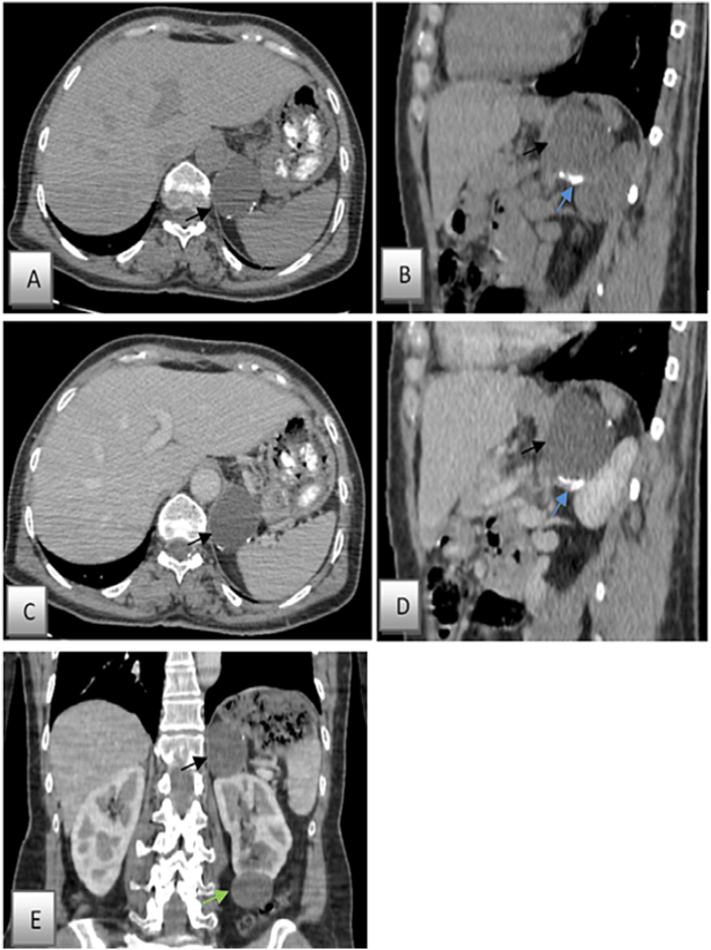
Non contrast-enhanced abdominal CT scan images (Fig. A: axial, Fig. B: sagittal), and non enhanced images (Fig. C: axial, Fig. D: sagittal, Fig.E: coronal), revealing a large well-limited cystic, homogeneous mass of the left adrenal gland (black arrow), with regular contours, containing some linear parietal calcifications (blue arrow), not septated, not enhanced after injection of contrast agent, without any rupture or intracystic hemorrhage. The mass measured 4.5 × 4.4 × 5 cm in cranio-caudal, antero-posterior and transverse dimensions and was abutting various parts of the gastrointestinal tract and the kidney.
